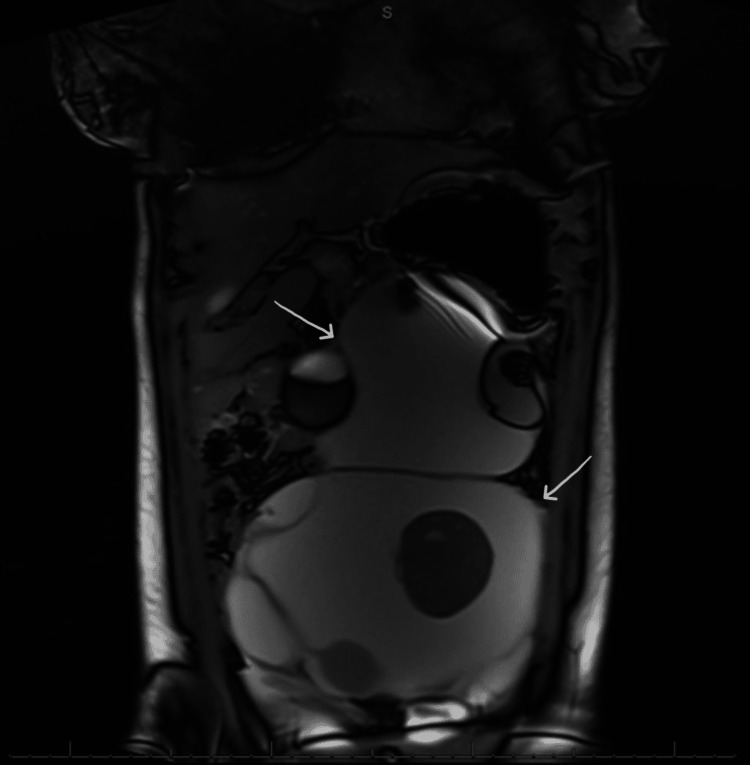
MRI of the abdomen with and without contrast demonstrating large central multilobed cystic structures with solid components, consistent with ovarian dermoid cysts (white arrows).
